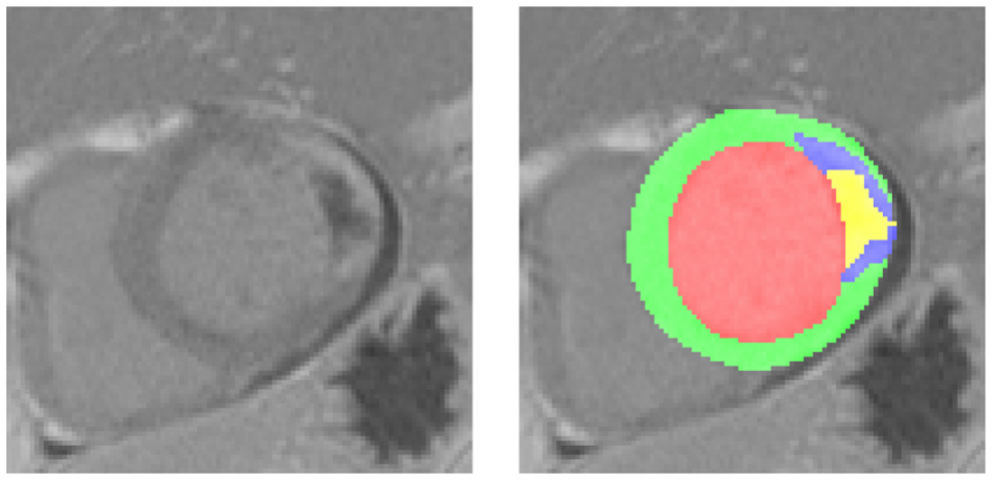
A typical Delayed Enhancement MRI (DE-MRI) slice involving the myocardial infarction (MI) and the persistent microvascular obstruction (PMO). On the left the cropped short-axis DE-MRI covering the whole left ventricle, on the right the corresponding masks of normal myocardium (green), MI (blue, PMO exclusive) and PMO (yellow). The PMO is semi-wrapped by the MI and contacts the cavity.
